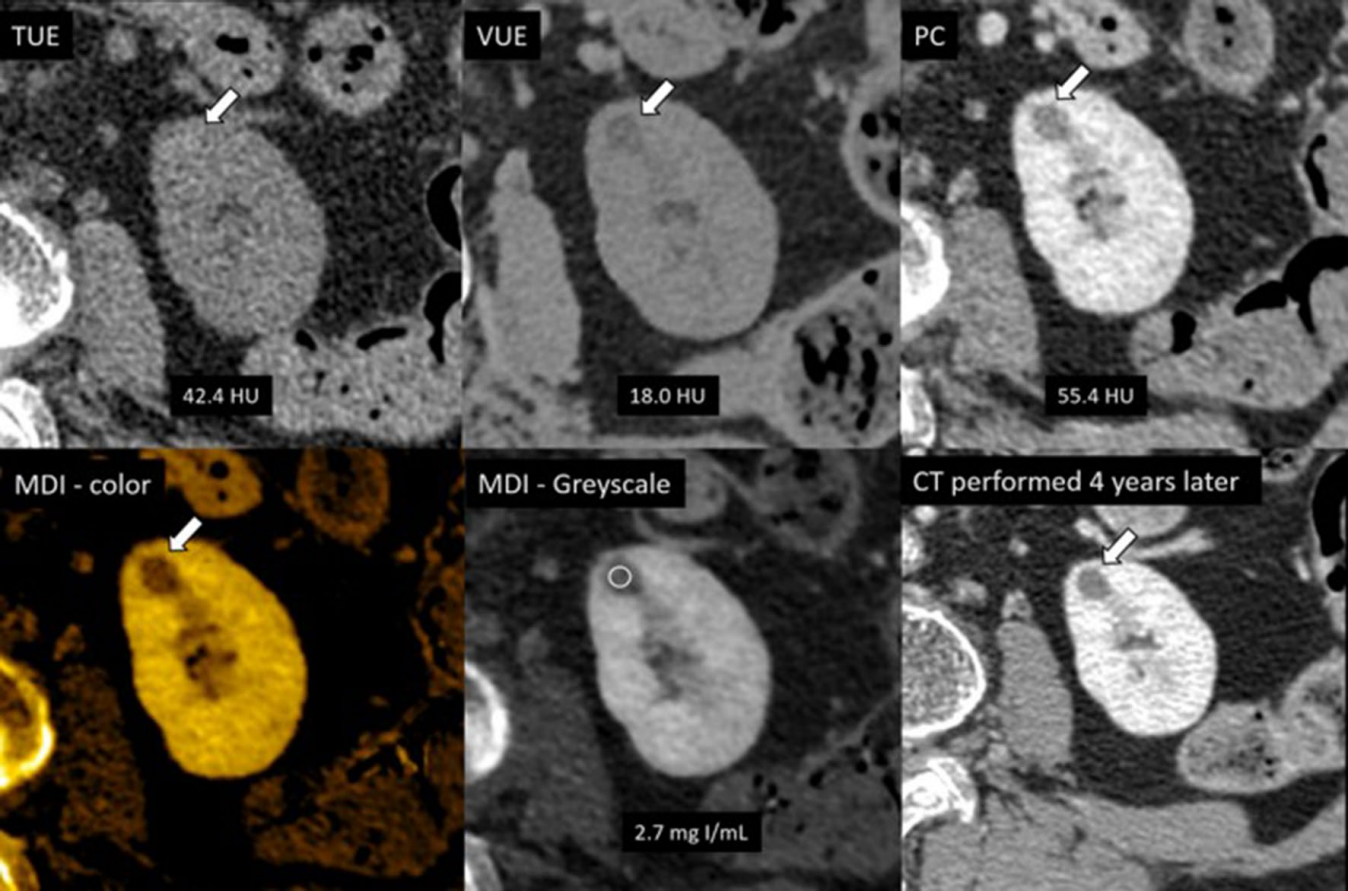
A 1.2 cm cyst with pseudoenhancement, misclassified as a mass based on iodine quantification (with an iodine concentration above the 1.6 mg I/mL cutoff). However, this lesion was correctly identified as a cyst on attenuation measurement and visual assessment of MDI images with high confident. This lesion was confirmed as a cyst by imaging criteria and has remained stable in size on CT imaging performed four years later. TUE = True unenhanced image, VUE = Virtual unenhanced image, PC = Post contrast images, MDI = Material density iodine images
